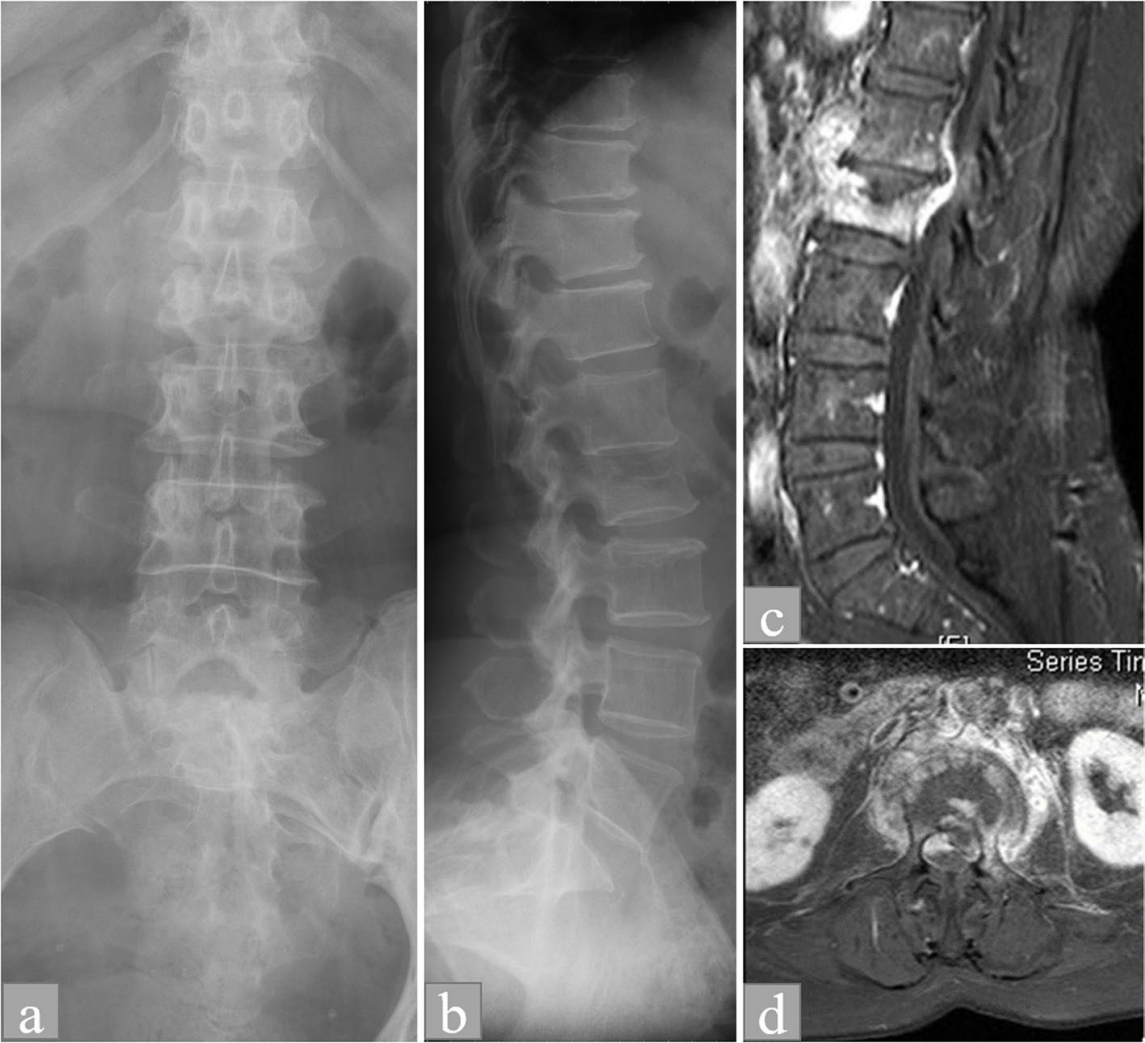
Anaerobic spondylodiscitis mimicking a fragile vertebral body compression fracture in a 72-year-old female (No. 3) with chronic lower back pain in the absence of fever. A Loss of vertebral body height without the widening of the interpedicular distance and interspinal process distance was observed at the L2 vertebra in the anteroposterior view of the lumbar spine radiograph. B Compression fracture of the L2 vertebra with preserved disk height was observed as an anterior wedging deformity and vertebral body height loss in the lateral and anteroposterior view of the lumbar spinal radiograph. C Heterogeneous enhancement of the L2 vertebral body and prevertebral space was observed on a sagittal gadolinium-enhanced fat-suppressed T1-weighted magnetic resonance image. D Enhancement of the paraspinal soft tissue muscle surrounding the L2 vertebral body. A spinal epidural abscess was observed as an epidural mass with central hypointensity and surrounding linear enhancement on an axial view of contrast-enhanced T1-weighted imaging
